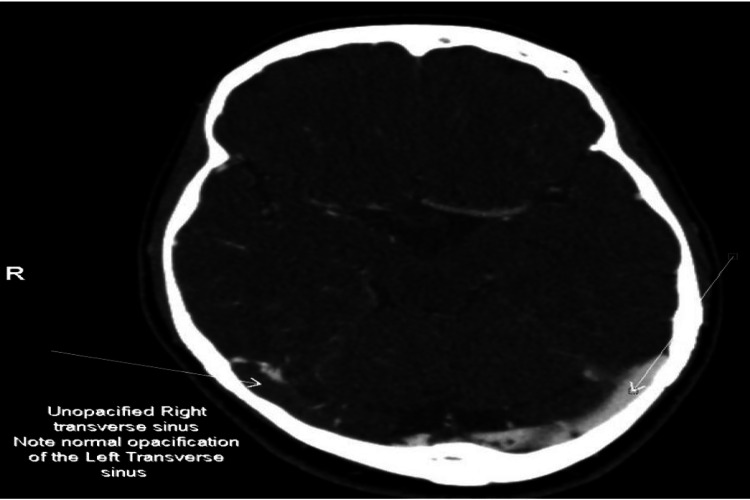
Axial view of the CT cerebral Venogram shows unopacified, thrombosed Right Transverse sinus.
